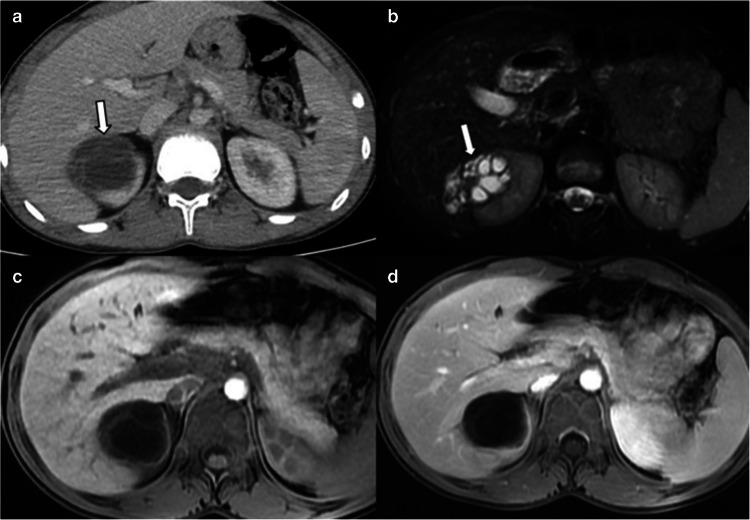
Type 2 renal hydatid cyst. A 15-year-old boy presented with right flank pain. a Axial contrast-enhanced computed tomography image reveals a multiseptated cystic lesion (*arrow*) in the upper pole of the right kidney, mimicking a neoplasm. b Axial fat-saturated T2-weighted image shows a multiloculated cystic lesion containing cysts of various sizes, compatible with daughter cysts (*arrow*). c, d Axial pre-contrast (c) and post-contrast (d) T1-weighted images demonstrate no internal vascularity. Serological tests were positive, and the lesion was treated by interventional radiology
